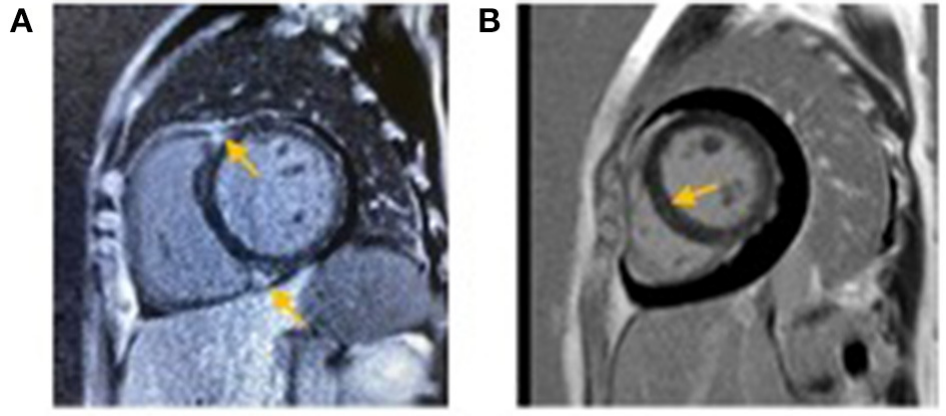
(A) Case 2 (Yellow arrow): Cardiac magnetic resonance imaging showing patchy delayed enhancement of the insertion at both ends of the ventricular septum. (B) Case 4 (Yellow arrow): Cardiac magnetic resonance imaging showing linear delayed enhancement in the basal segment of the ventricular septum.
